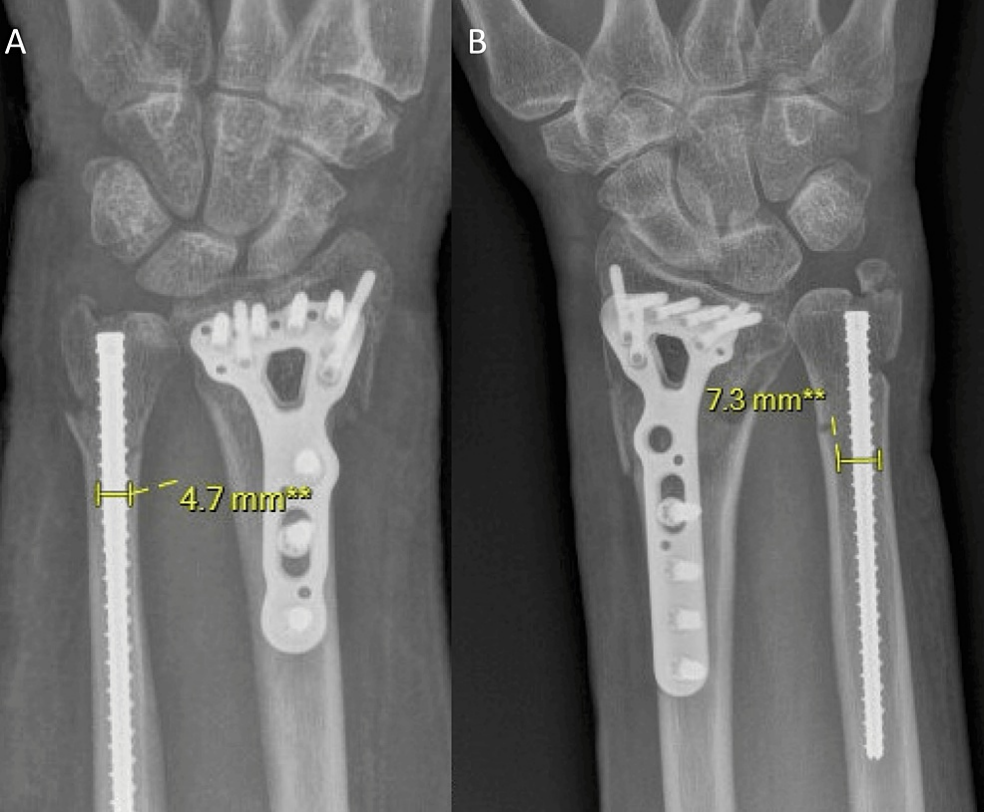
From left to right; post-operative AP radiographs of Case 2 (A) and Case 3 (B), respectively, demonstrating the distal ulna metaphyseal isthmus diameter, which was the width of the intramedullary canal measured 3 cm from the ulnar head articular surface.
